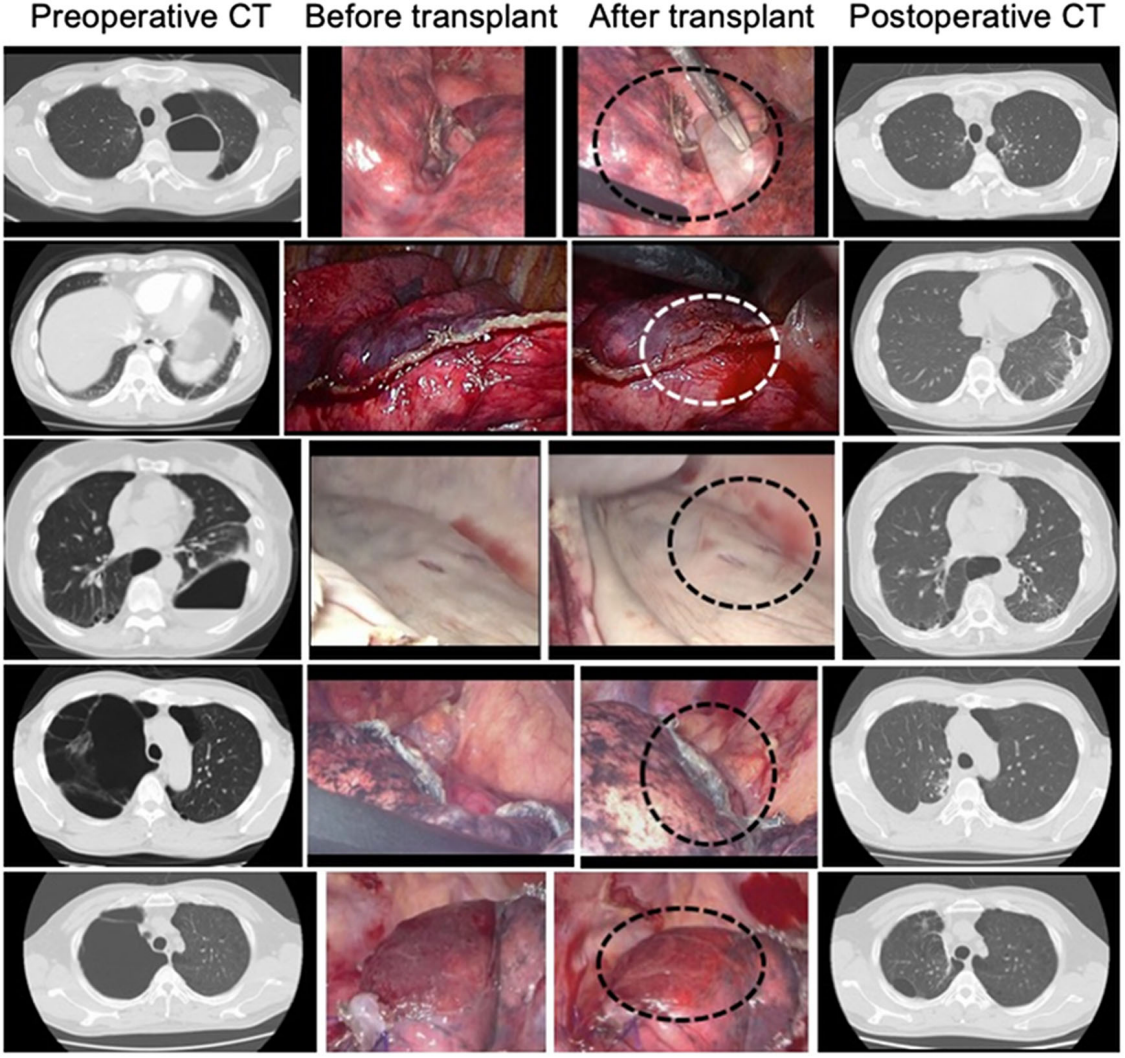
Chest computed tomography and intraoperative findings. Left, middle, and right columns of photographs represent preoperative chest computed tomography (CT), before fibroblast sheet transplantation from intraoperative digital video data, after fibroblast sheet transplantation from intraoperative digital video data, and postoperative chest CT, respectively. From case 1 to case 5 in this order from the upper row. The area of air leaks is surrounded by dashed lines in the middle column.
